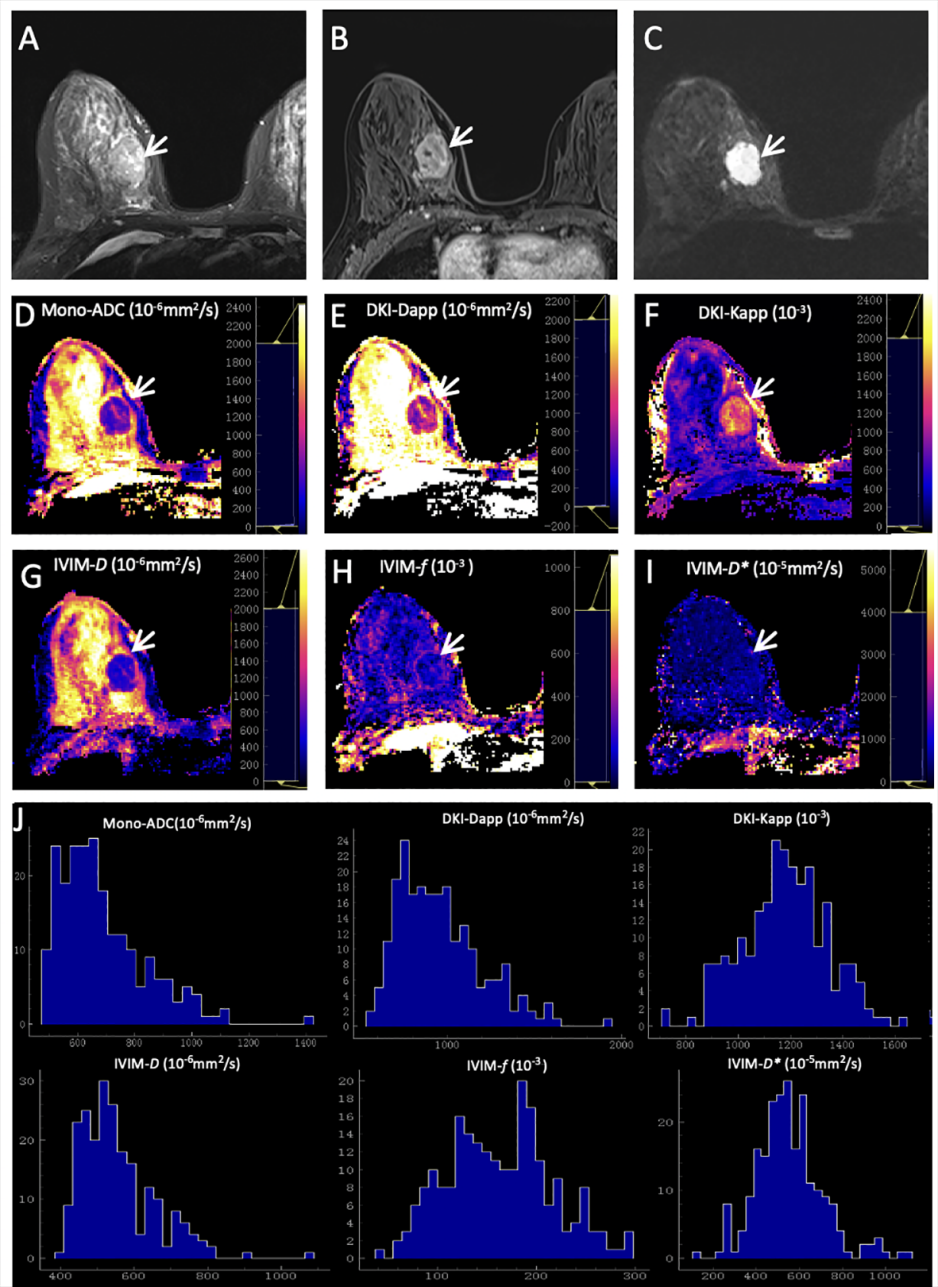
Representative images of a grade 2 invasive ductal carcinoma in the right breast of a 42-year-old woman. This tumor was with positive expression of estrogen receptor (95%) and progesterone receptor (20%), high Ki-67 (40%), and negative HER2 expression. (A) T2WI. (B) This mass is oval and with obvious enhancement (arrow) on axial DCE-MRI. (C) This mass shows oval shape, smooth margin, and homogeneous signal on DWI (b-value = 1000 mm^2^/s) (arrow). ADC (D), Dapp (E), Kapp (F), *D*
